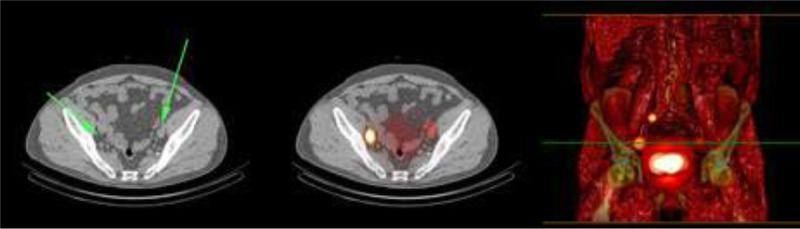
On the left, CT image of the same area revealed no pathologic enlarged lymph node at the right side (arrow right). At the left side, an enlarged lymph node is seen (arrow left). In the middle, transaxial fused SPECT/CT image of lymphatic drainage with SN seen along the iliac artery at both sides. On the right, volume-rendered fusion image of both scintigraphy SPECT and CT is seen with focal uptake in the SN along the right iliac artery. At the left side, a contralateral SLN is seen (crossover phenomenon). At the right side, a higher echelon node is seen along the common iliac artery.
